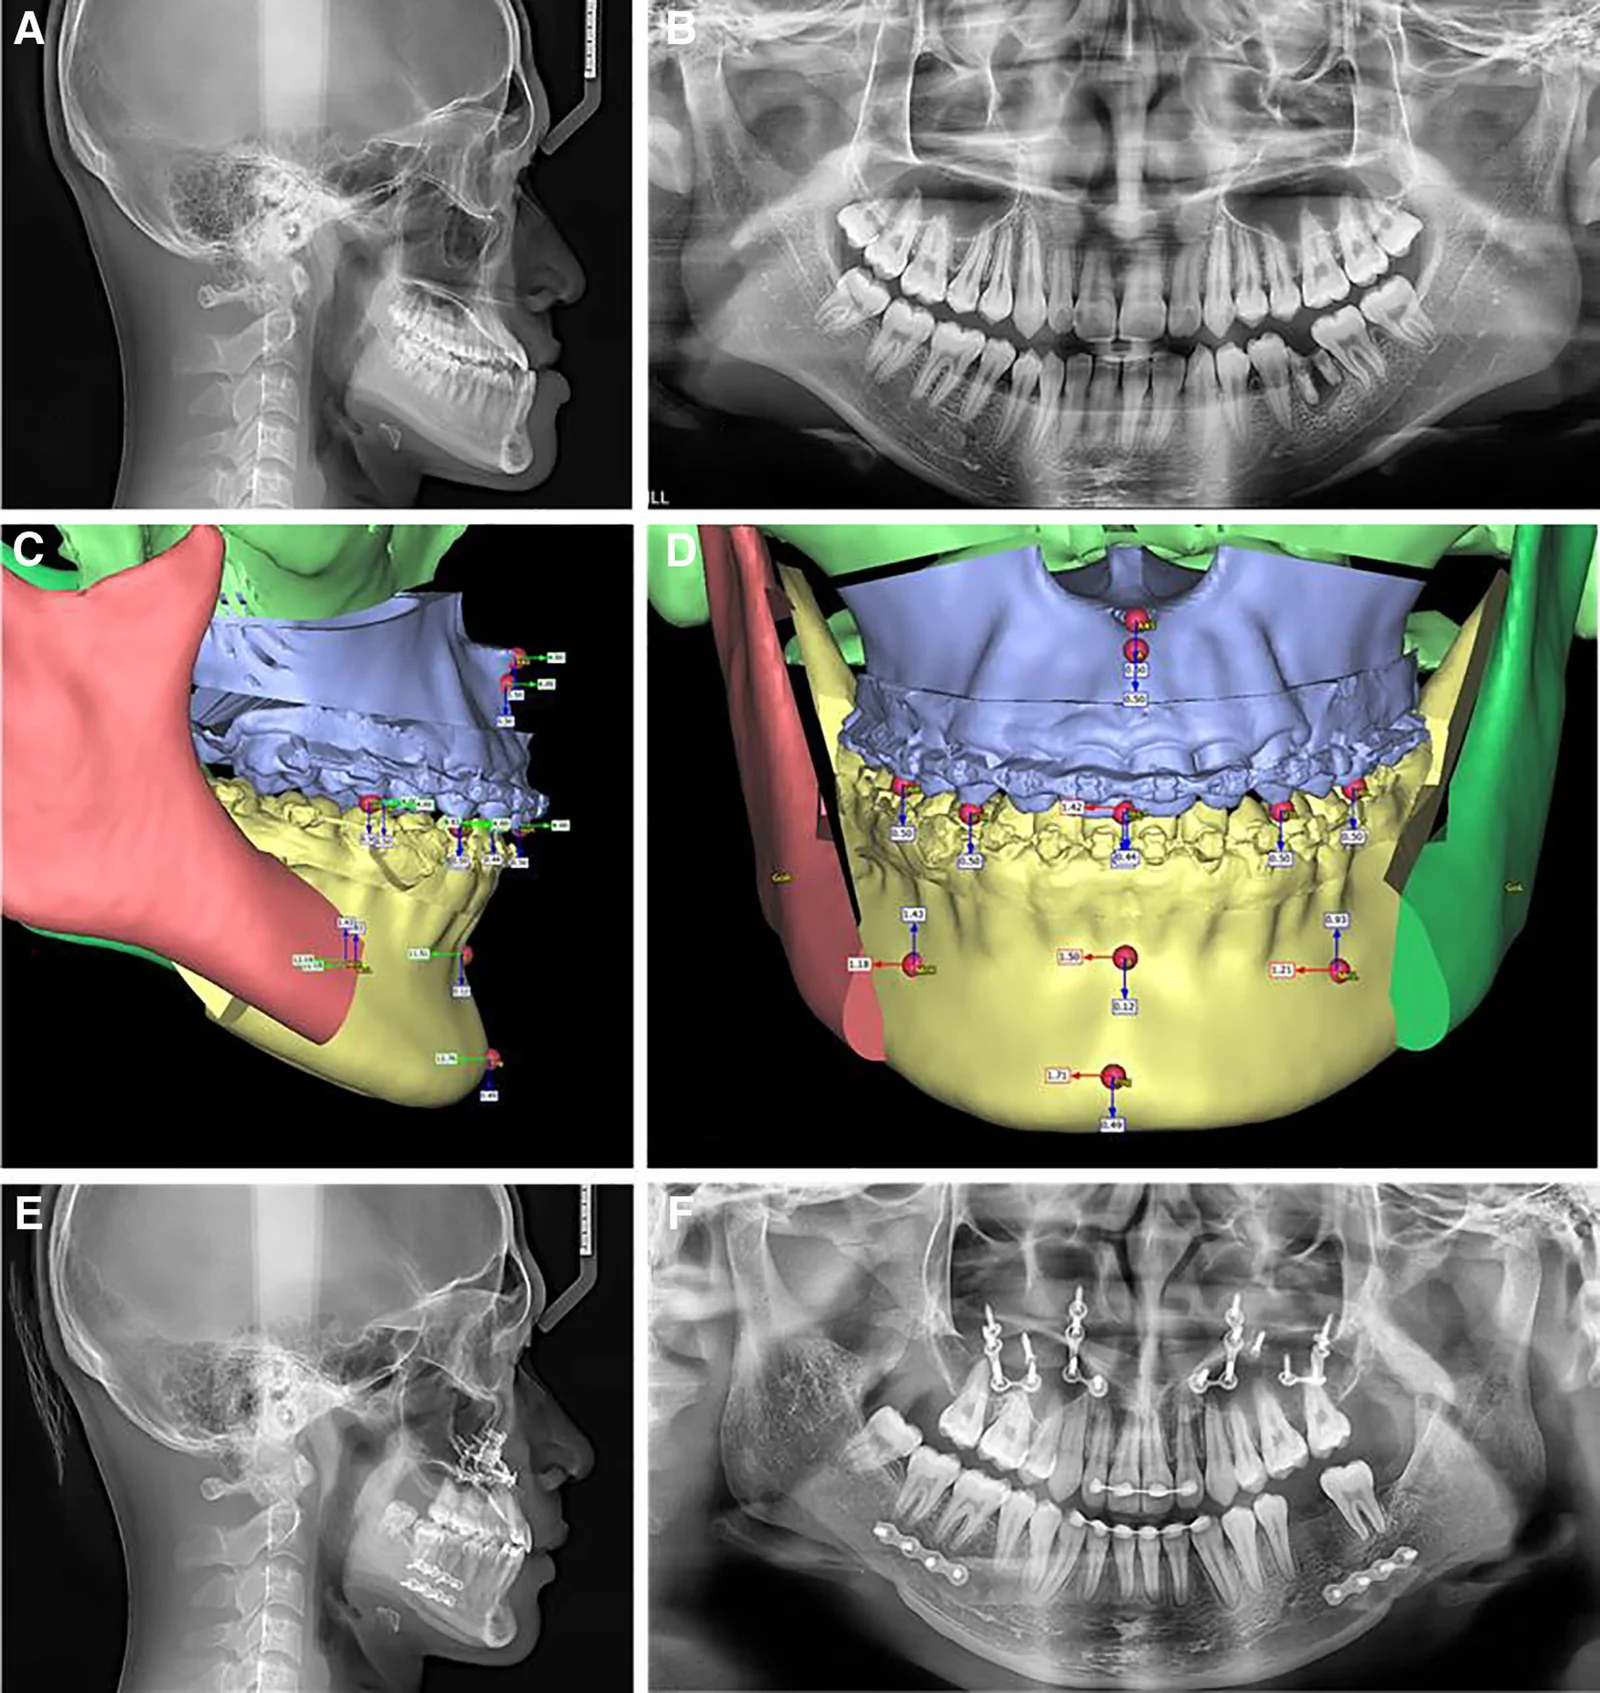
Case 2, radiographs. (A, B) Pretreatment. (C, D) Surgical plan. (E, F) Posttreatment.
